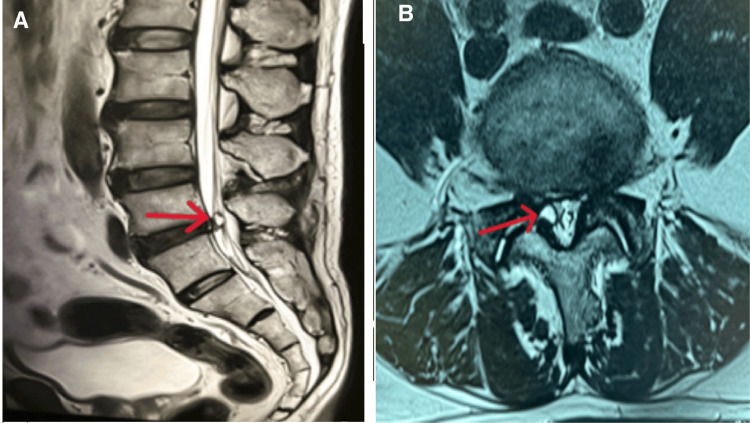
T2-weighted MRI images (A) Sagittal view of the lumbosacral region demonstrating a lumbar facet joint synovial cyst measuring 4.1 x 4.3 x 6.5 mm (red arrow) within the spinal canal. (B) Axial view of the lumbar region at L4/L5 vertebral level demonstrating the cyst (red arrow) lying anteromedially to the degenerated right L4/L5 facet joint, causing severe spinal canal stenosis and exit foraminal stenosis.
